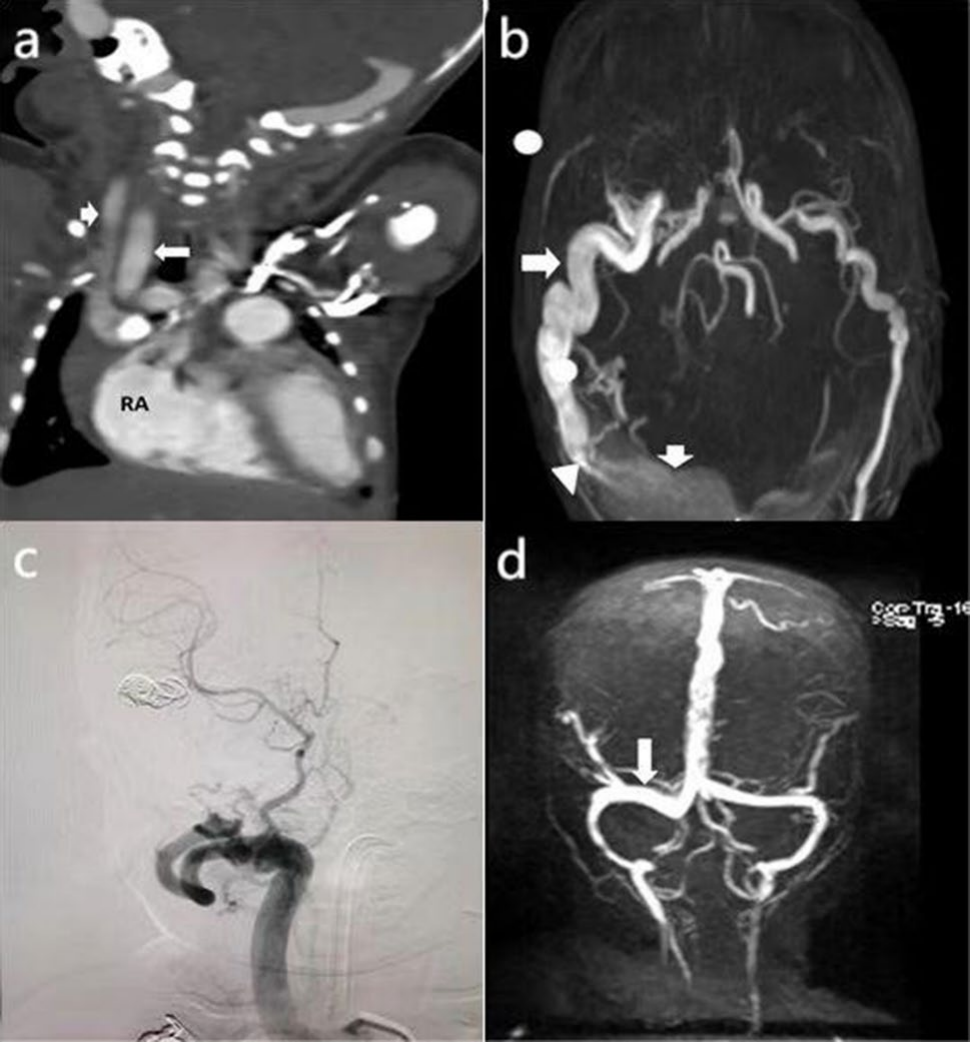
a Computed tomography: enhanced coronal view of the heart. The right carotid artery and jugular vein are dilated, and the right atrium is enlarged. Long arrow, right external carotid artery; short arrow, right external jugular vein; *RA* right atrium. b Head magnetic resonance angiography. The dilated right dural artery and dilated venous sinus form an arteriovenous fistula. Long arrow, right dural artery; short arrow, right transverse sinus; triangular arrow, arteriovenous fistula. c Digital subtraction angiography after the first embolization. Abnormal shunts, such as the arteriovenous fistula between the right dural artery and the dilated venous sinus, disappeared after embolization. d Re-examination 4 months after the second operation. Magnetic resonance venography shows that the dilated right transverse sinus has become normal. Long arrow, right transverse sinus
